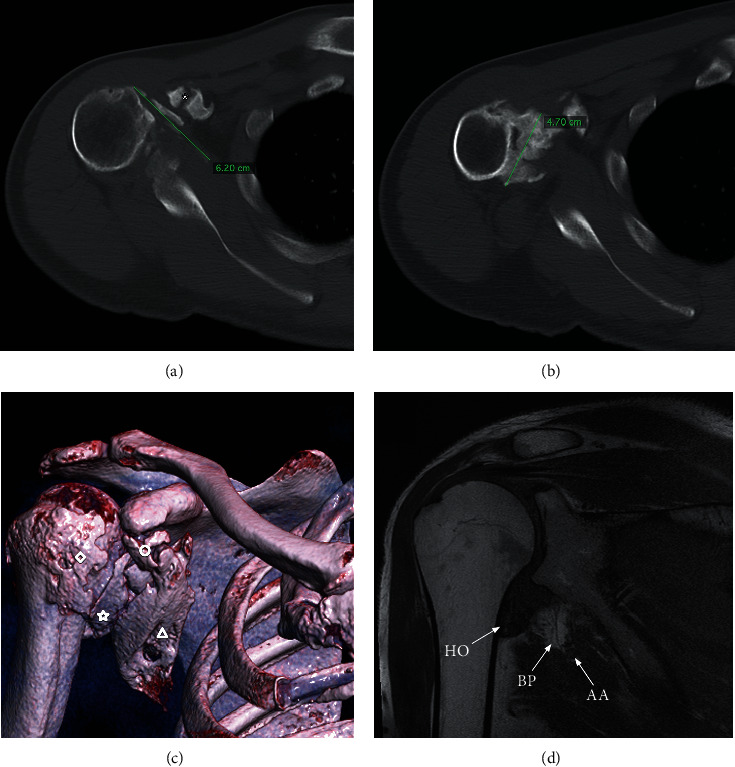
(a–d) 9 months after traumatic anterior dislocation, preoperative CT and MRI images demonstrating severe HO. (a) HO along the bony insertion and ventral side of the subscapularis muscle (green line) and around the conjoint tendons (asterisk) in the axial CT plane. (b) HO extending from the inferior glenoid to the proximal humeral shaft and axillary recess in axial CT plane. (c) 3D CT scan showing the extent of HO, primarily in the ventral part of the subscapularis muscle (triangle), in the axillary recess (asterisk), conjoint tendons (circle), and ventrally of the proximal humerus (diamond). (d) MRI demonstrating close location of the HO (HO) and both axillary artery (AA) and nerves of the brachial plexus (BP).
